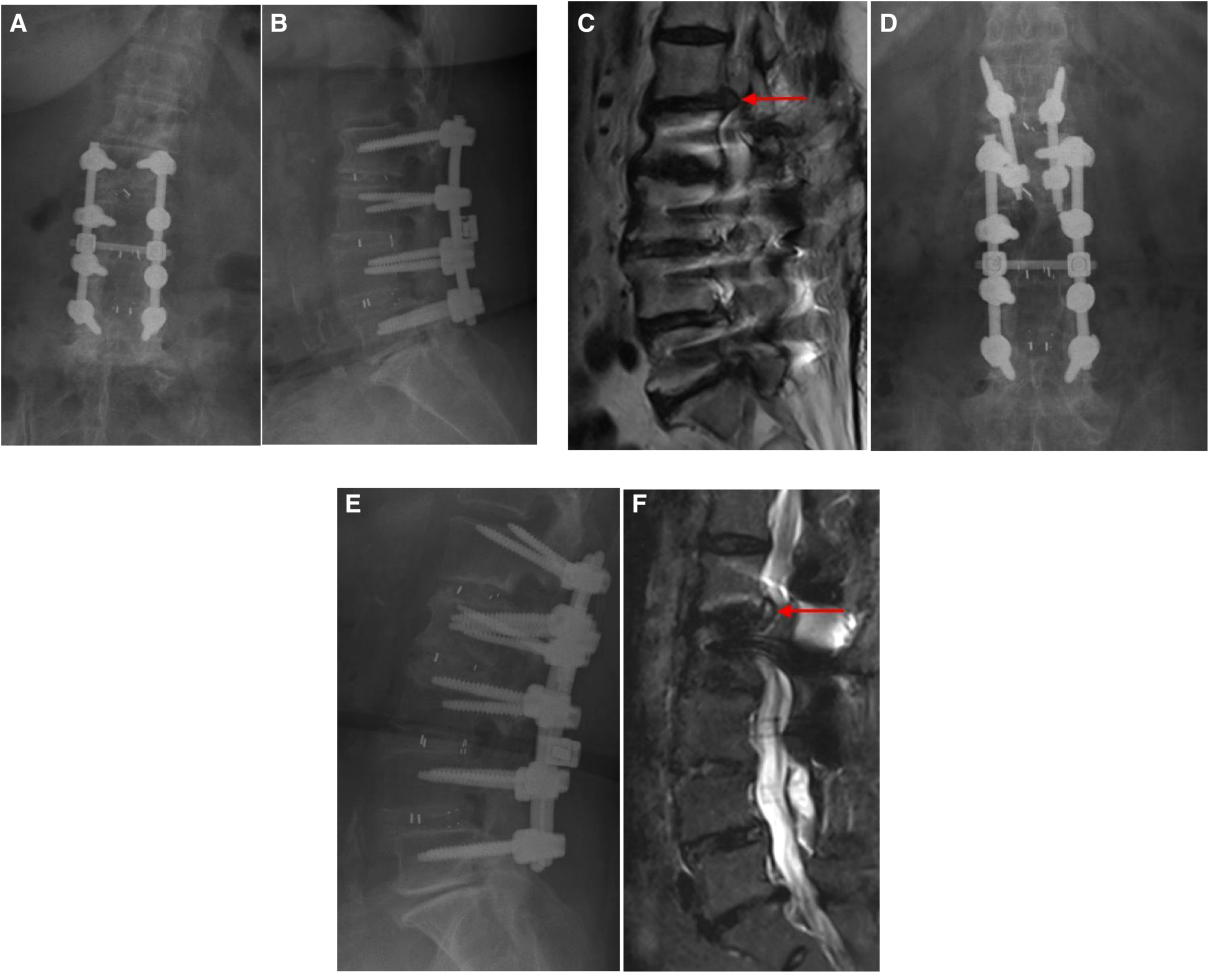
(A, B) A patient underwent lumbar 2/3 and L3/4 and lumbar 4/5 TLIF combined with pedicle screw fixation 4 years ago, and numbness and pain recurred in his left thigh 1 year ago. (C) MRI of the lumbar spine has shown that lumbar disc herniation was observed at the superior segment adjacent to the fusion segment (L1/2 level), as demonstrated by the red arrows. (D, E) Minimally invasive interbody fusion combined with CBT screws fixation was performed at L1/2. (F) MRI has demonstrated that the compressed nerve roots were decompressed, as demonstrated by the red arrows. TLIF, transforaminal lumbar interbody fusion; MRI, magnetic resonance imaging; CBT, cortical bone trajectory screw; L1/2, interverbal disc space between lumbar segments 1 and 2.
